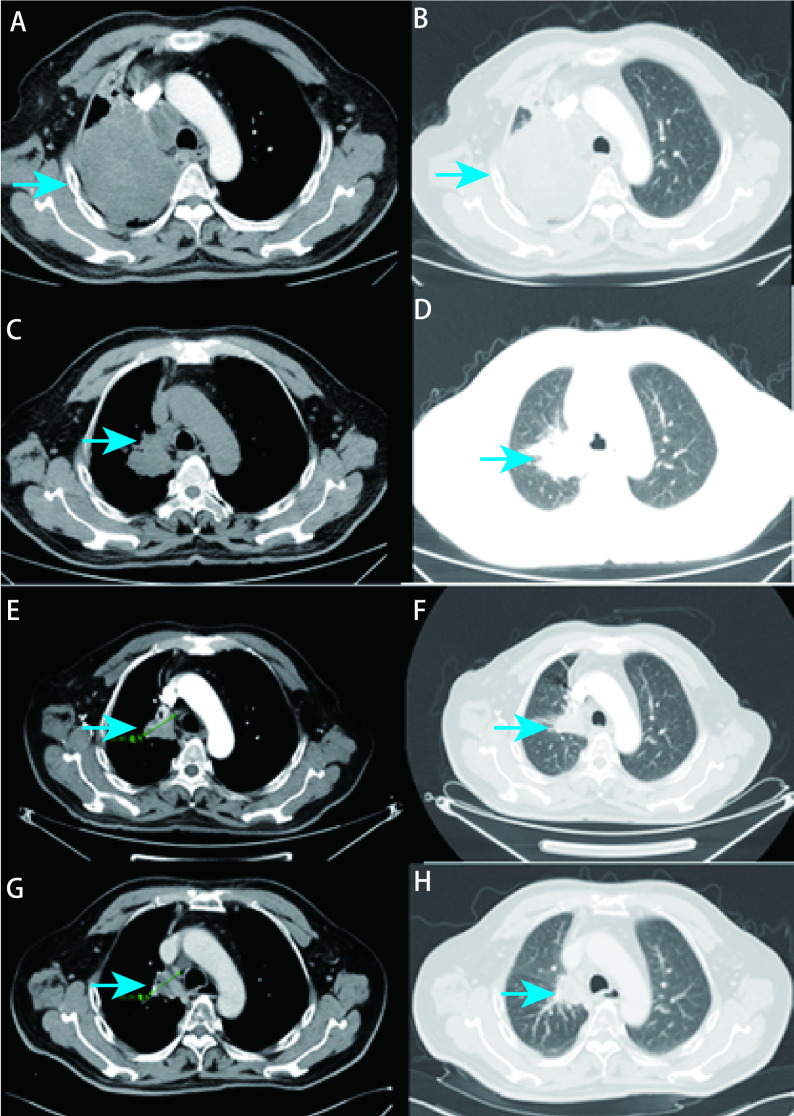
病例2在诊断和治疗中不同时间节点的胸部CT表现。A、B：患者确诊时的胸部CT表现，右肺上叶占位，病灶大小为104 mm×85 mm（A：纵隔窗；B：肺窗）；C、D：靶向治疗后，肺部占位较前缩小，大小为50 mm×40 mm（C：纵隔窗；D：肺窗）；E、F：肺部占位较前增大，出现颅脑转移，评价为PD（E：纵隔窗；F：肺窗）；G、H：确诊为小细胞肺癌，4个周期EP化疗后，肺部占位较前略减小（G：纵隔窗；H：肺窗）。 Case 2 chest CT findings at different time points during diagnosis and treatment. A, B: Chest CT findings of the patient at the time of diagnosis, occupying in the upper lobe of the right lung, lesion size 104 mm×85 mm (A: mediastinum window; B: lung window); C, D: After targeted therapy, the lung space was reduced to 50 mm×40 mm (C: mediastinum window, D: lung window); E: Lung mass increased and brain metastasis appeared, which was evaluated as PD (E: mediastinum window; F: lung window); G: Diagnosed as small cell lung carcinoma, the lung space occupied decreased after 4 cycles of EP chemotherapy (G: mediastinum window; H: lung window). EP: Etoposide+Carboplatin. PD: progressive disease.
